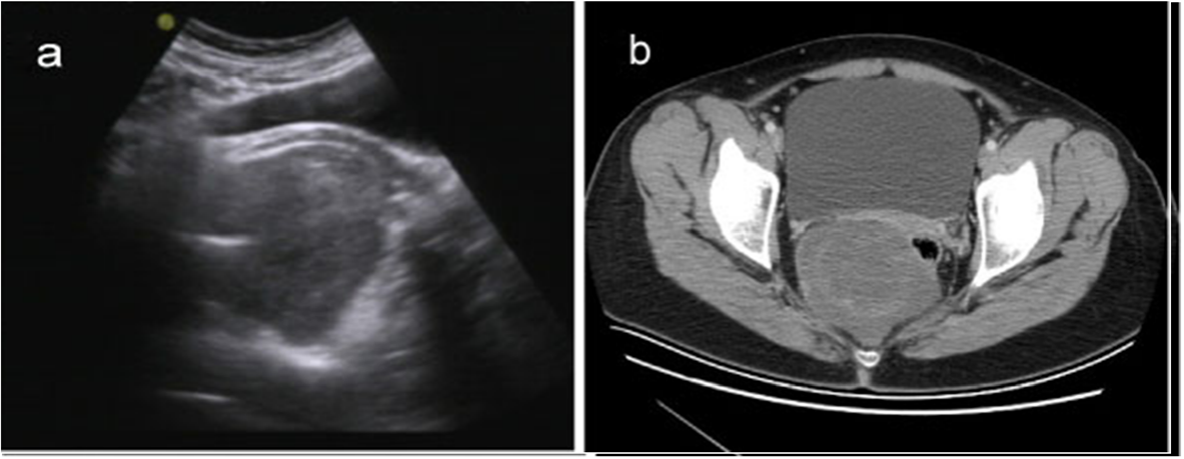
Image feature of tumor pre-operation. (a) Transvaginal ultrasonography shows an oval, moderately echoic mass in close proximity to the cul-de-sac of Douglas. (b) Pelvic CT scan shows a well-circumscribed tumor of homogeneous intensity located anteroinferior to the sacrum.
